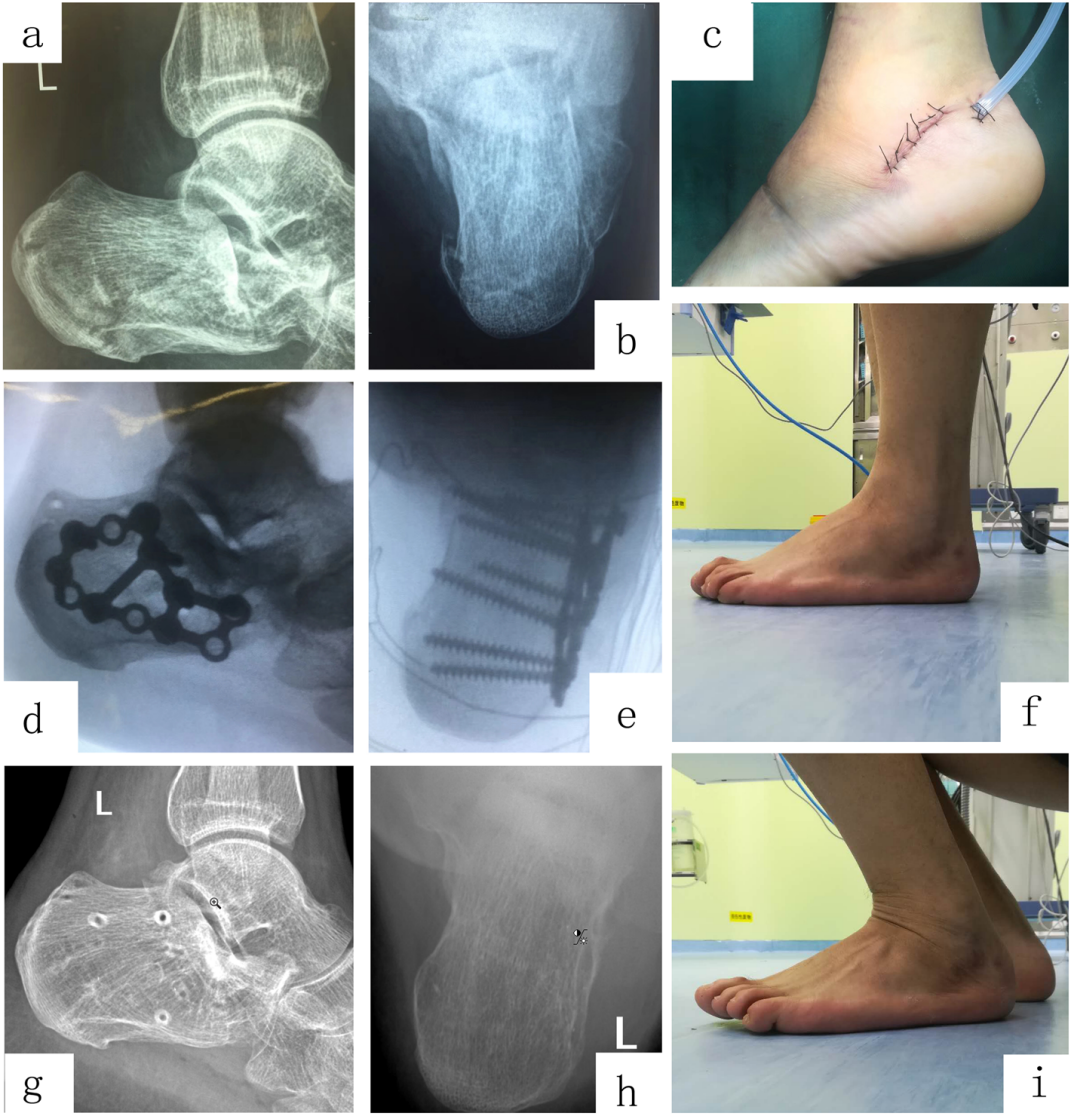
A 43-year-old male patient falls from a height of 3 m. A preoperative lateral radiograph (a) showing right calcaneus fracture with reduced Böhler angle (9°), length (81.9 mm), and height (70 mm), and the preoperative horizontal (b, c) computed tomography image showed Sanders type IIA fracture with increased calcaneal width (39 mm). The Steinmann pin retractor (d, e) was used during the operation. Postoperative lateral (f, g) and axial (h) radiographs show obvious correction of the Böhler angle (34°), and improvements in calcaneal length (88.8 mm), height (77.5 mm), and width (33 mm). AOFAS score was 89 at the last follow-up
